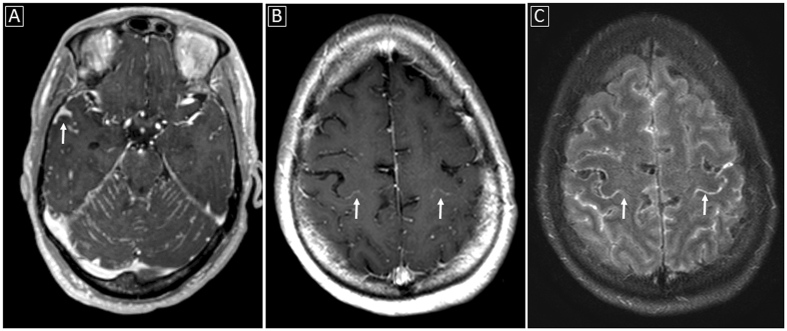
Examples of meningeal involvement in CNS cryptococcosis: (A) Abnormal meningeal enhancement along the cerebellar folia as well as along﻿ the sulci within the middle cranial fossae (white arrow) on enhanced T1-weighted image; (B and C) Sulcal meningeal enhancement especially delineating the central (white arrows) and postcentral sulci on enhanced T1-weighted and enhanced FLAIR images obtained near the convexity.
